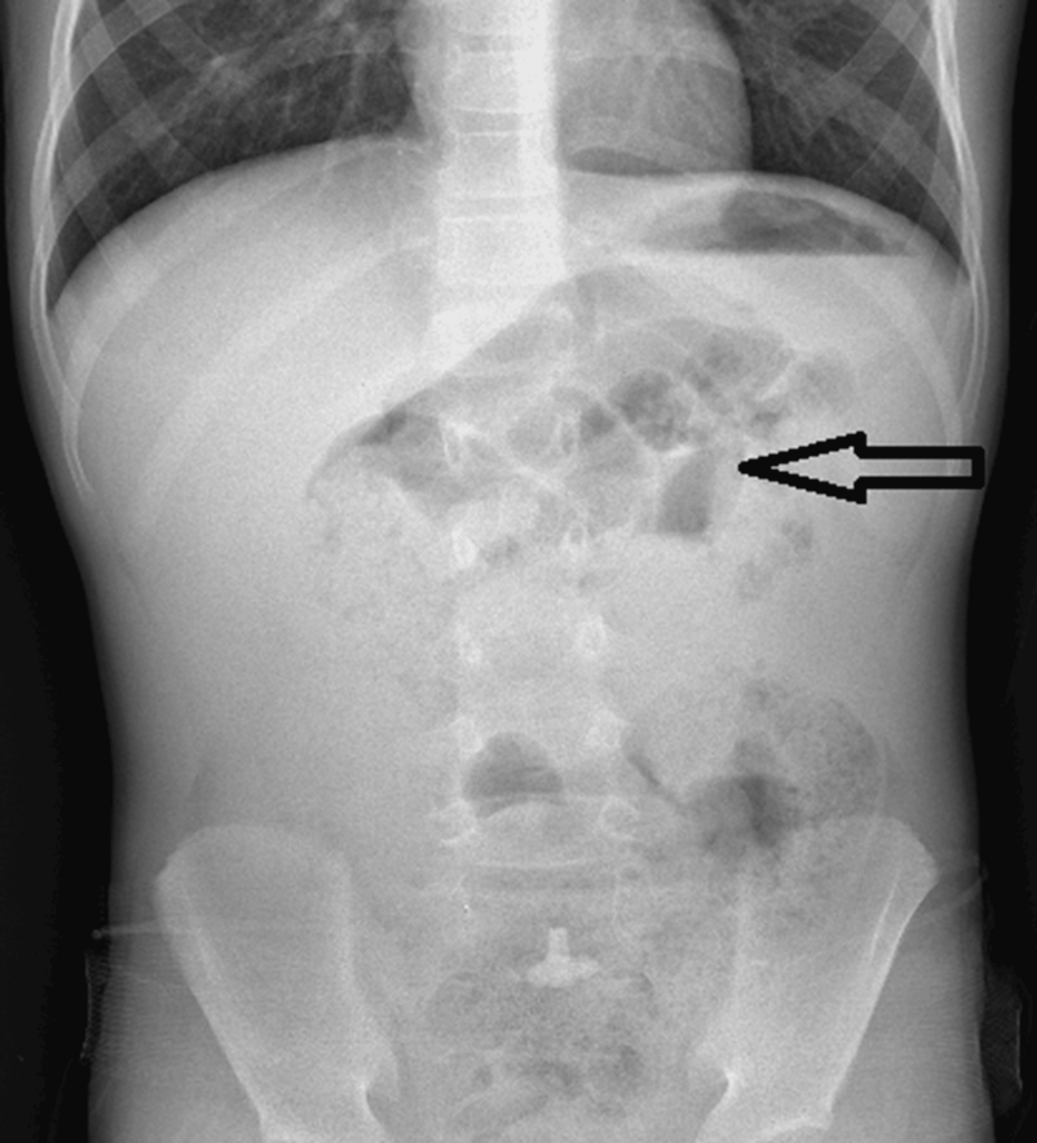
X-ray of the abdomen and pelvis showing multiple air fluid levels (arrowhead). Arrowhead showing multiple air fluid levels.
